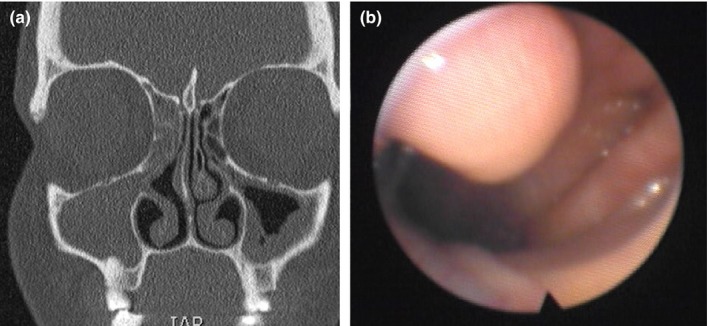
CT Scan and nasofibroscopy reporting posterior middle turbinate. The coronal plan of CT Scan showed a chronic rhinosinusitis of both maxillary sinuses A, The nasofibroscopy of the left nasal cavity exhibited a mass in the nasopharyngeal cavity.
